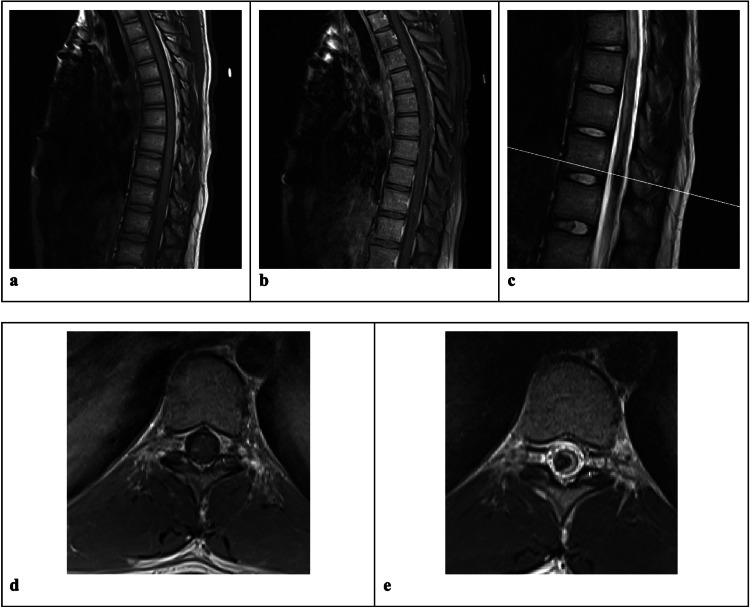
Imaging at presentation Sagittal T1 MRI (a) and sagittal T1+ MRI with contrast (b) demonstrate enhancement of an intramedullary spinal cord lesion. Sagittal T2 MRI (c) demonstrates the presence of hyperintensity cranial and caudal to the lesion, suggesting edema. Axial T1 MRI (d) and axial T2 MRI (e) reveal lateralization of the lesion to the right.
